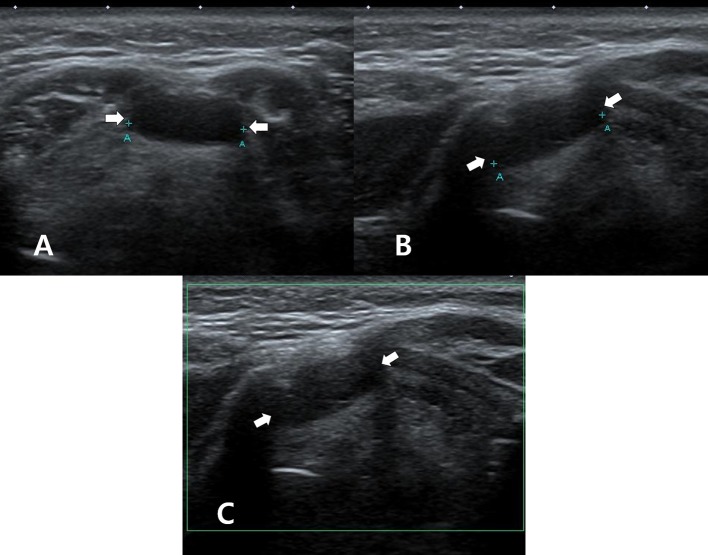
A 50~55-year-old woman who underwent thyroid ultrasonography (US) owing to health screening but had no history of thyroid surgery or radioactive iodine ablation. On thyroid US, transverse gray-scale (A), longitudinal gray-scale (B), and longitudinal color Doppler (C) US images show a well-defined cystic lesion with a flat-to-ovoid shape in the suprahyoid, anterior upper midline neck (arrows, 1.3 cm in length).
